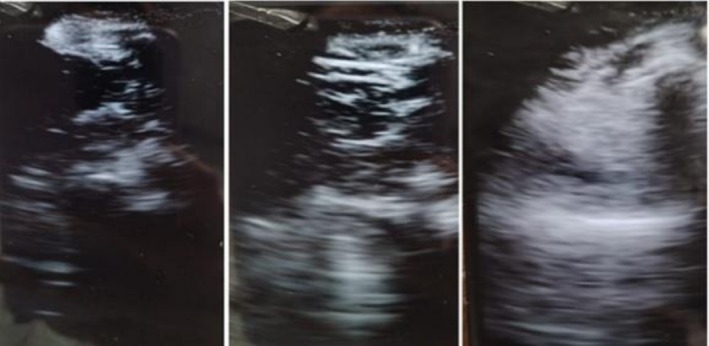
Patient 2. Left and center: Ultrasound of the left lung base in posterior view, showing several foci of intraparenchymal infiltrates. Right: Anterior fields of the right lung base, showing a large intraparenchymal infiltrate.
